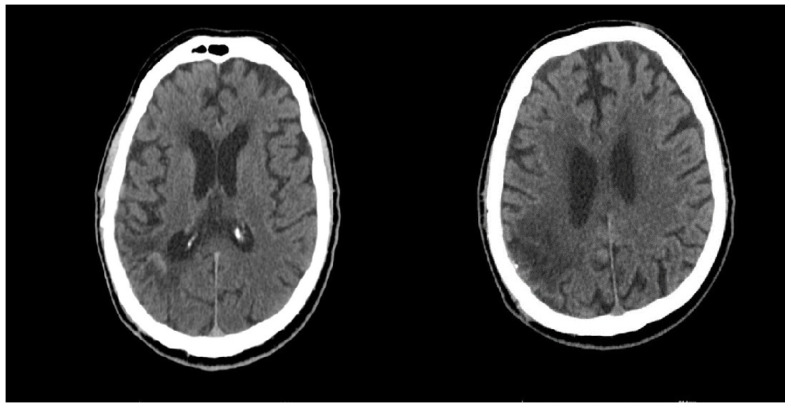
One year post-surgery, a contrast-enhanced CT shows no sign of tumor recurrence.
